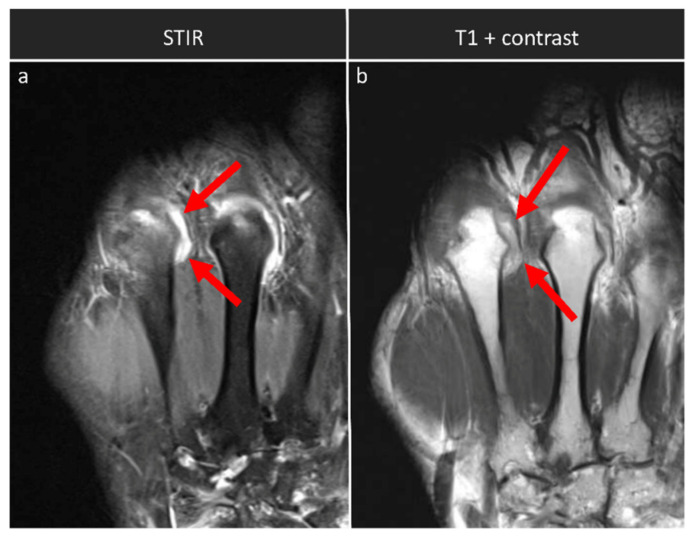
Synovitis in an exemplary patient across the sequences. This coronal section of a STIR sequence (a) and a T1-weighted post-contrast sequence without fat suppression (b) visualizes synovitis at joint effusion at the ulnar aspect of the MCP II joint of the right hand of a 51-year-old female patient. Synovial thickening and effusion as signs of synovitis are visible in both sequences (red arrows in (a,b)).
